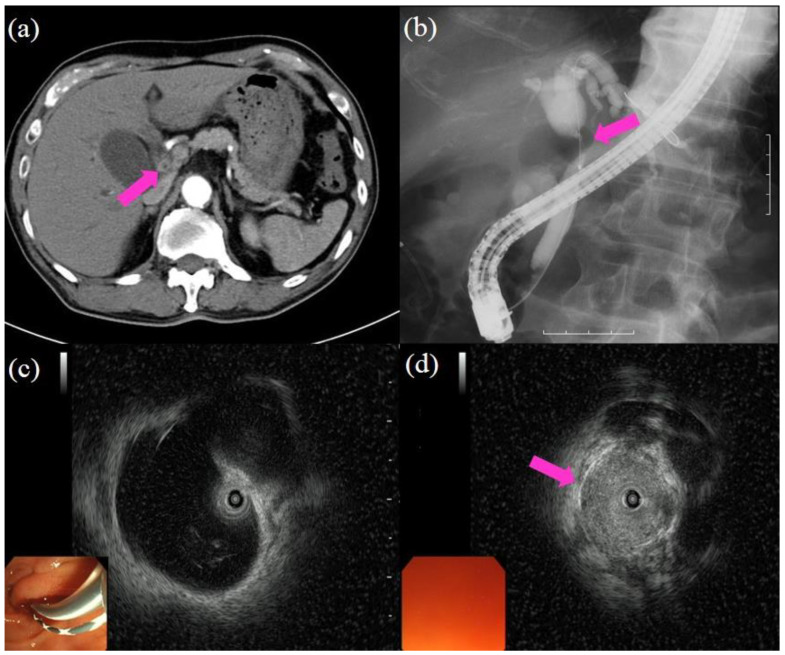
Procedures of cholangiography and intraductal ultrasound (IDUS): (a) computed tomography showing the wall thickness in the bile duct (pink arrow); (b) cholangiography showing the biliary stricture in the hilar bile duct (pink arrow); the proximal part of the bile duct shows dilatation; (c) IDUS showing dilatation in the proximal part of the bile duct and no lesion; (d) IDUS showing a lesion in the biliary stricture (pink arrow).
